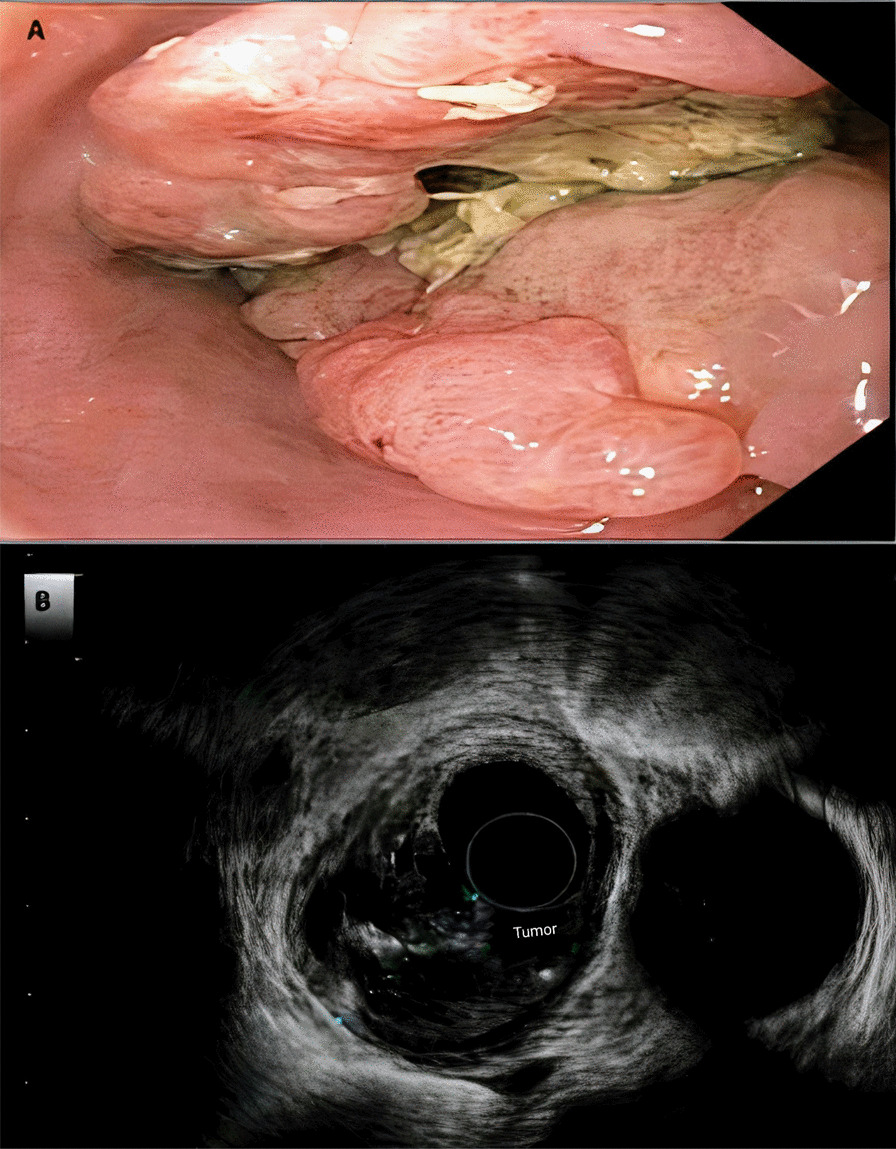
A Esophagogastroduodenoscopy and B endoscopic ultrasound showing the tumor
